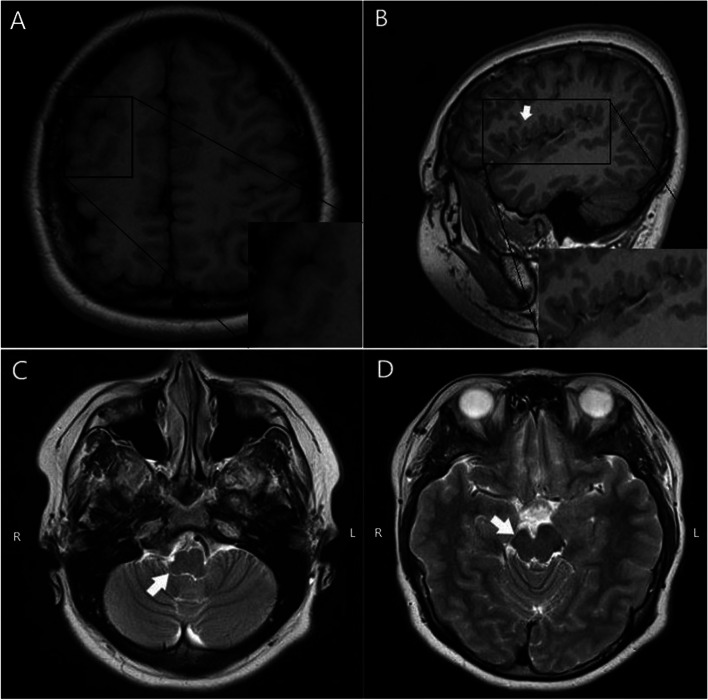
The axial T1-weighted image shows irregularities of the gray–white matter junction, and the cortex displays a nodular, bumpy appearance (A, arrow). MRI shows that the right half of the brain was smaller than the left half by visual comparison (A). The sagittal image reveals multiple small gyri in the sylvian fissure (B, arrow). The axial T2-weighted images show that the right half of the pons was significantly smaller than the left half (C, arrow), and the right cerebral peduncle of the midbrain was significantly smaller than the left (D, arrow)
